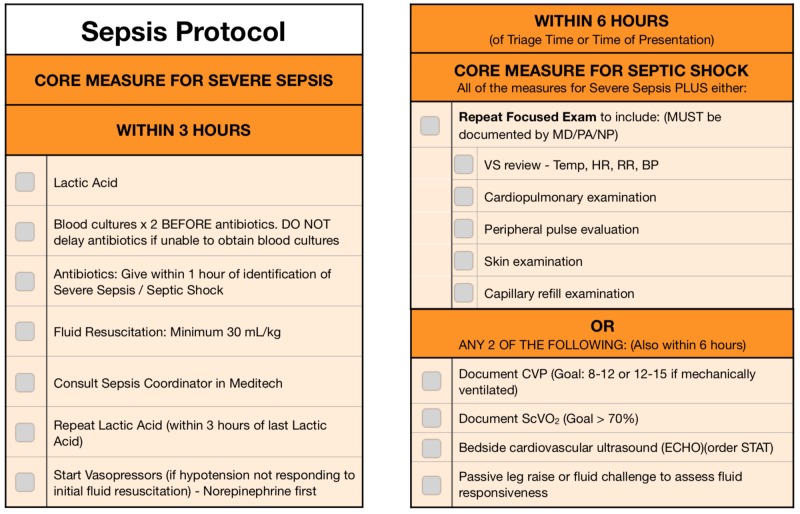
Sepsis Card MD = Doctor of Medicine; PA = Physician Assistant; NP = Nurse Practitioner; HR = Heart Rate; RR = Respiratory Rate; BP= Blood Pressure; CVP = Central Venous Pressure; ScVo2 = Central Venous Oxygen Saturation ECHO= Echocardiography
